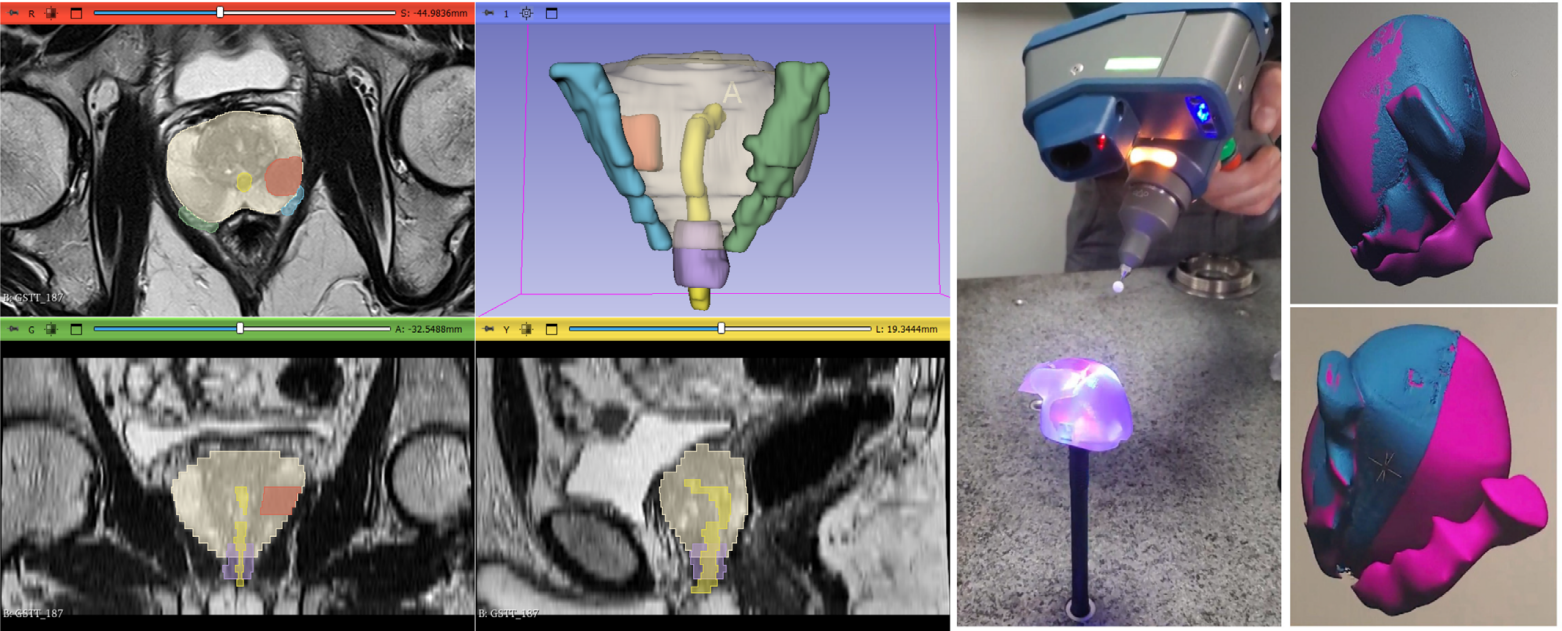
Left: segmentation of patient‐specific prostate anatomy on multiparametric MRI (mpMRI) to extract annotations of the prostate gland (shown in translucent white), tumour lesions (shown in red), external sphincter (shown in purple), urethra (shown in yellow), and neurovascular bundles (shown in blue and green). Right: annotations resulting from segmentations are three‐dimensional (3D) printed and scanned using a coordinate measuring machine that captures a 3D representation of the physical model. The overlapping of annotations (in pink) and scanned 3D representation (in blue) validates that 3D printed models and 3D virtual models fully correspond to each other.
